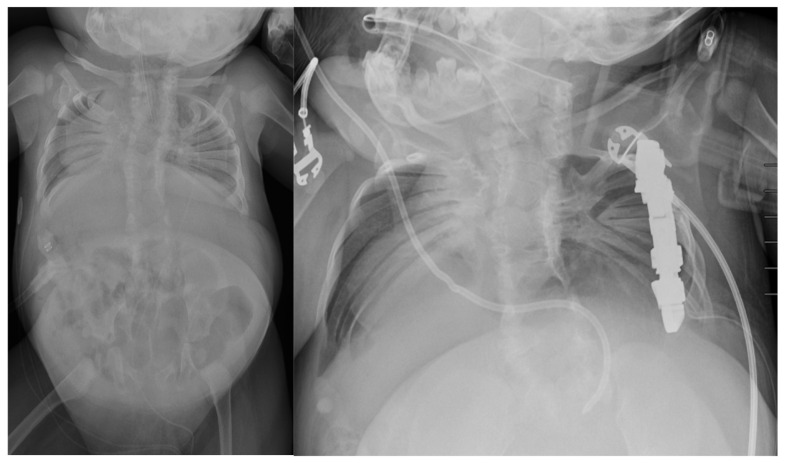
Pre- and post-right rib wedge osteotomy and VEPTR insertion radiographs demonstrating the bilateral fusion of the costovertebral junction in a patient with STD.
